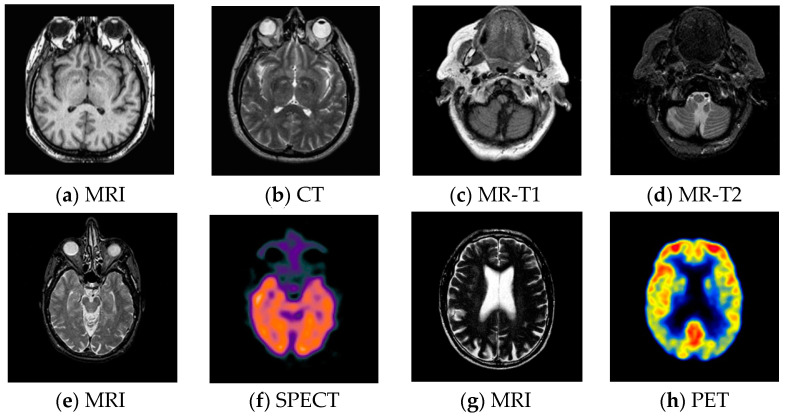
Pairs of medical source images: (a,b) MRI/CT images, (c,d) MR-T1/MR-T2 images; (e,f) MRI/SPECT images; (g,h) MRI/PET images.
